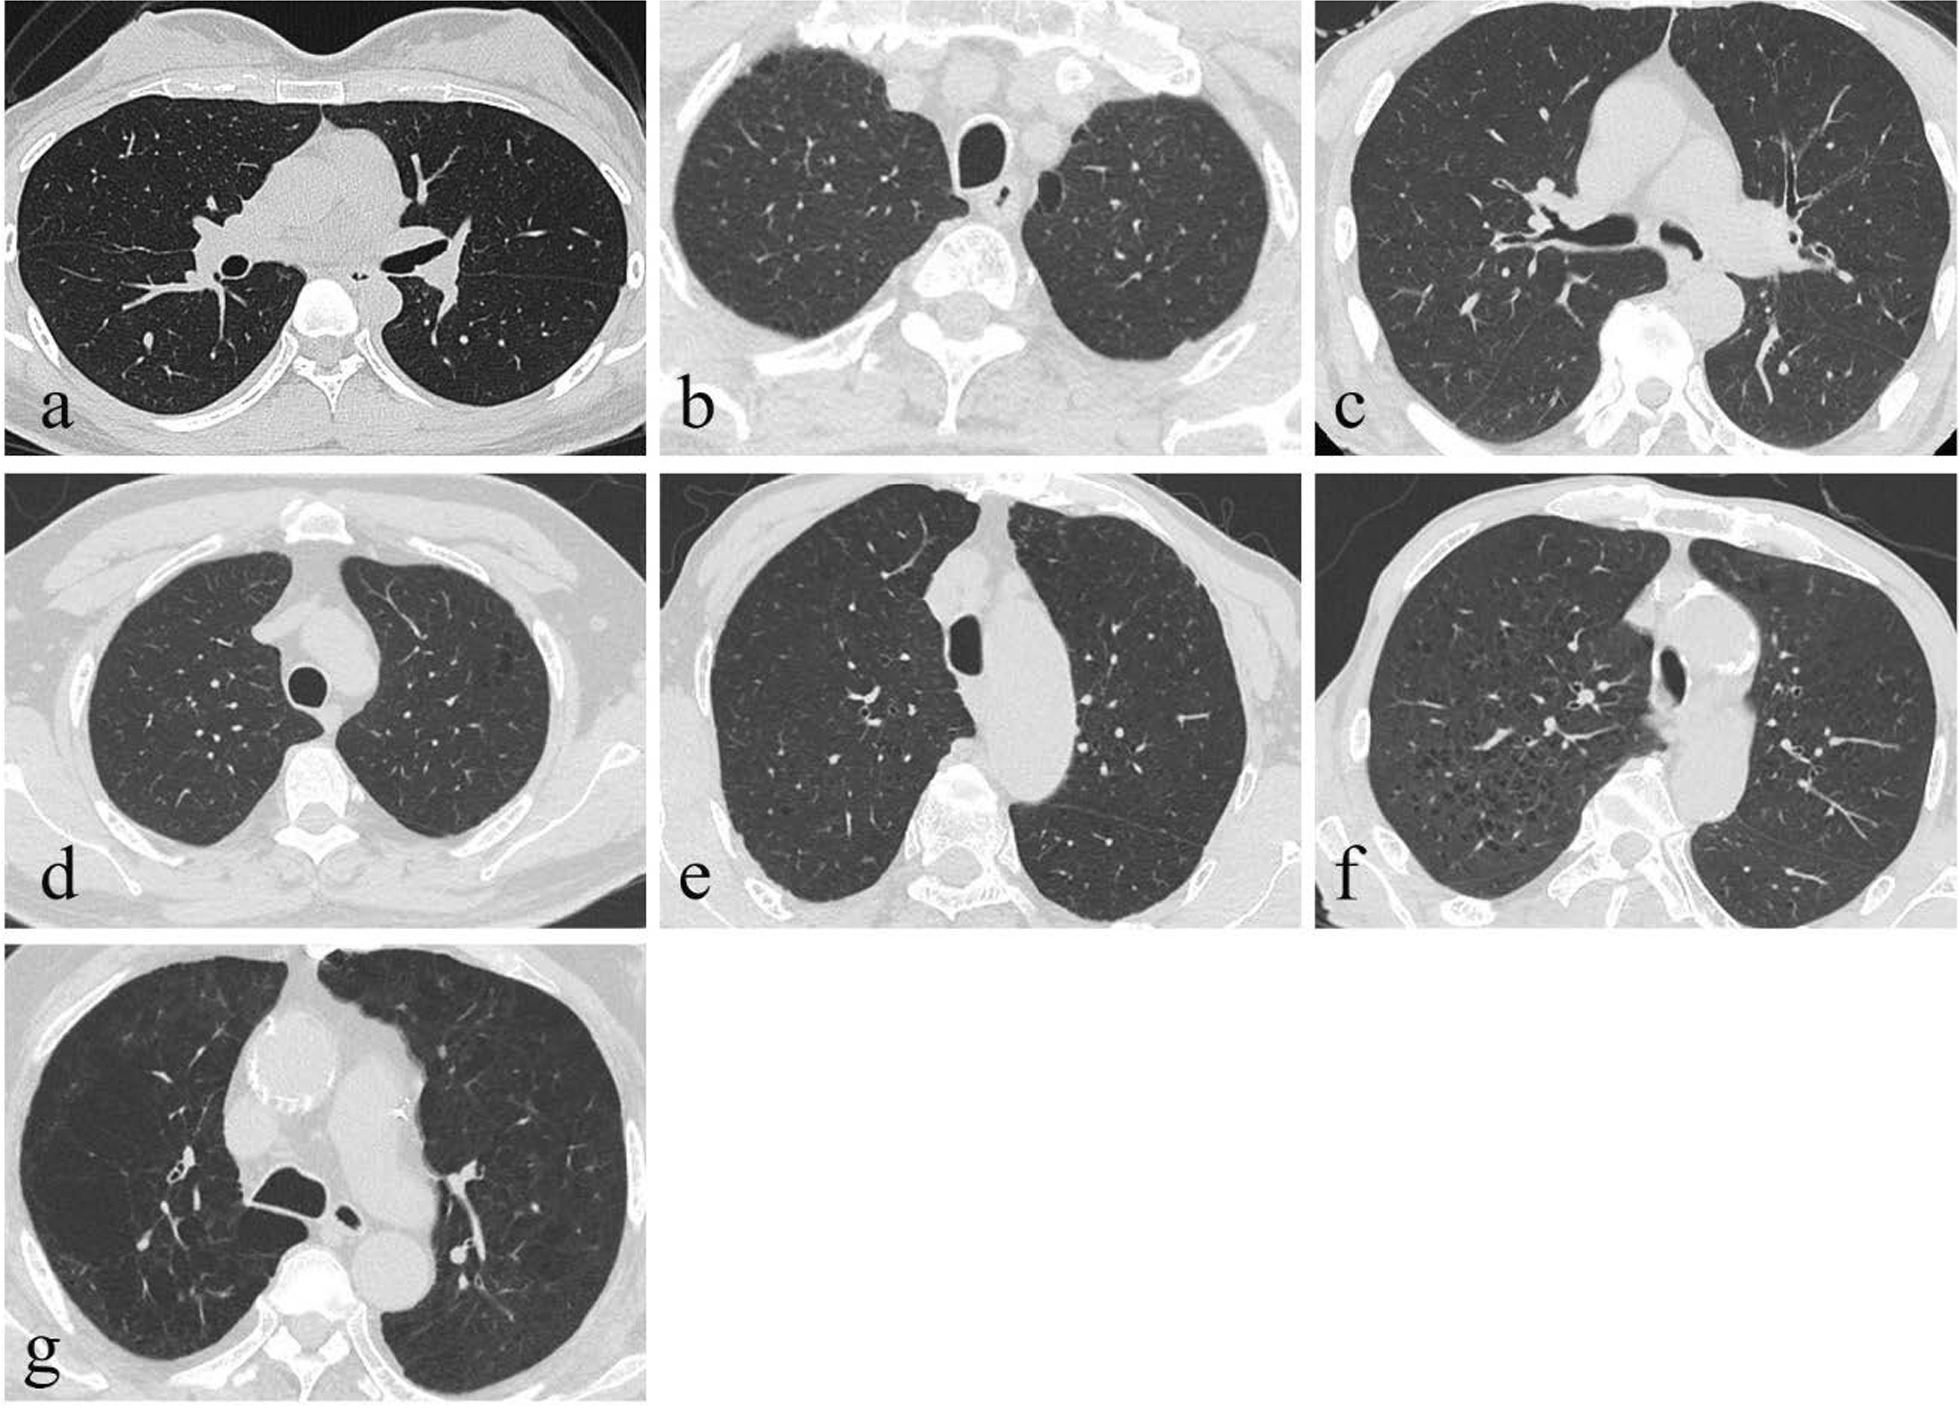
HRCT manifestations of seven different visual subtypes. a Normal. b Paraseptal emphysema. c Bronchitis; HRCT shows thickening of the bronchial wall without obvious emphysema area. d Centrilobular emphysema (mild); LAA accounts for less than 0.5%. e Centrilobular emphysema(moderate); LAA accounts for 0.5–5%. f Centrilobular emphysema (severe); LAA accounts for more than 5%. g Advanced destructive emphysema; Panlobular emphysema with bronchus hyperexpansion and distortion
